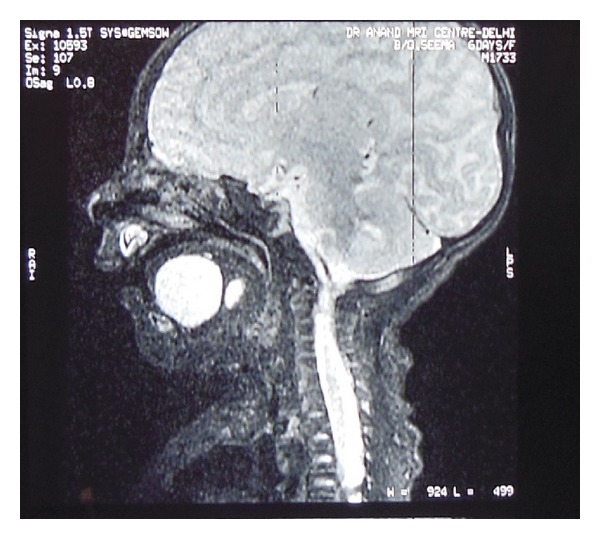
MRI scan: midline sagittal fat suppressed T2 weighted image showing the cystic lesion above the mylohyoid with no extension.
